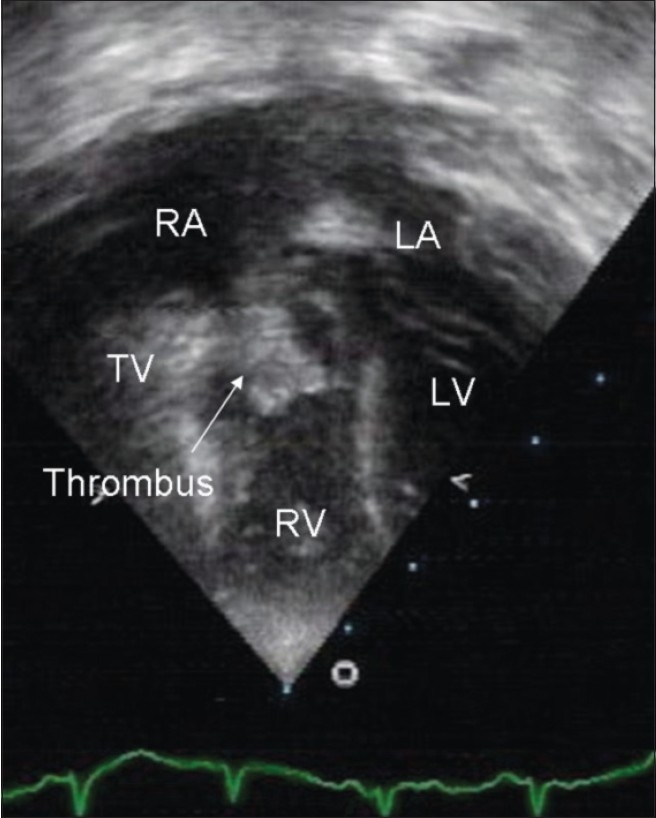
Two-dimensional echocardiography, a four chamber view showing thrombus going through the tricuspid valve in diastole LA: Left atrium, LV: Left ventricle, Ao.: Aorta, RA: Right atrium, RV: Right ventricle
